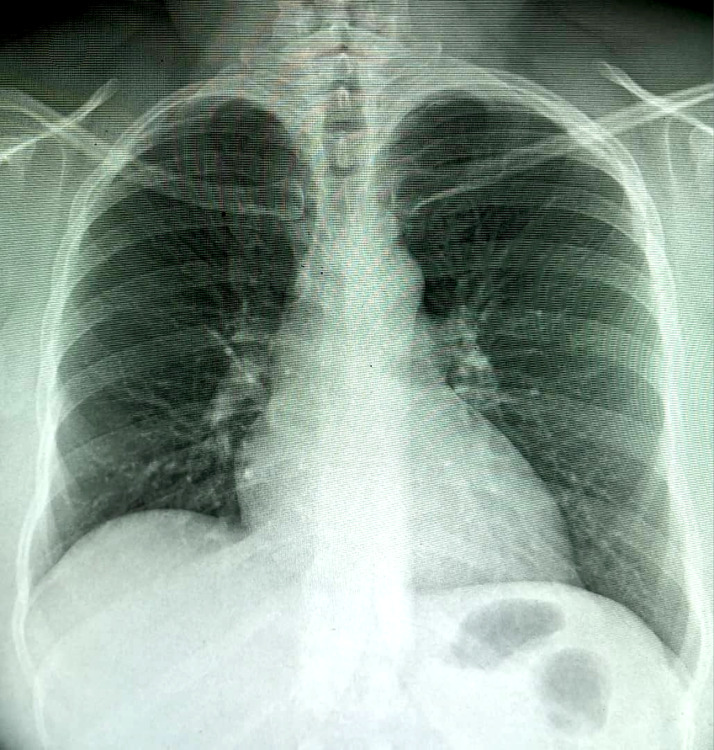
Radiographie du thorax de face: accentuation de la trame bronchovasculaire surtout au niveau de la base pulmonaire droite et cardiomégalie modérée avec index cardiothoracique à 0,60 Frontal chest x-ray: accentuation of the bronchovascular pattern more at the level of the right pulmonary base and moderate cardiomegaly with cardiothoracic ratio at 0.60
